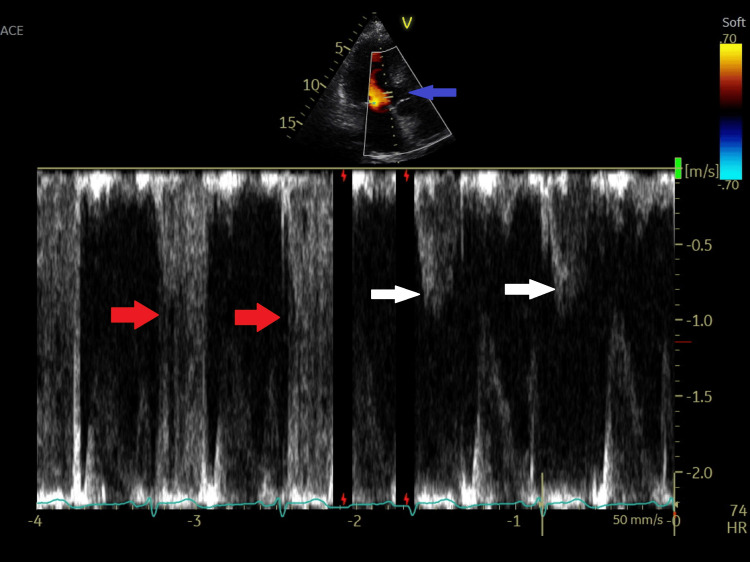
Pulsed Doppler wave recording from the posterior left ventricular outflow tract (red arrow) to the anterior LVOT ventricular outflow tract showing aliasing velocity in the posterior LVOT and normal velocity at the anterior LVOT (white arrow). The blue arrow indicates the final placement of the pulsed sample volume at the anterior LVOT.
